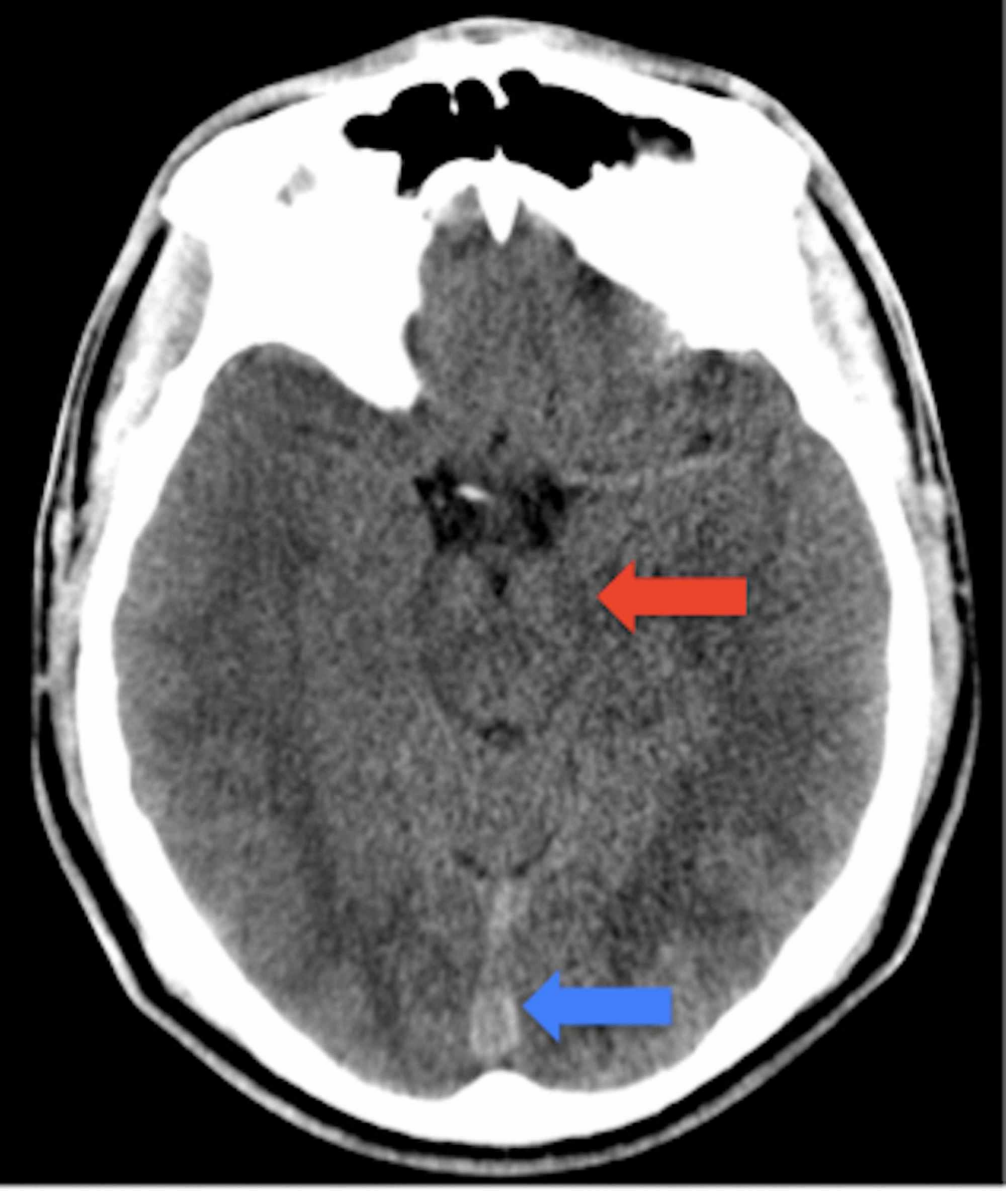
Noncontrast axial CT scan of the head at the level of the suprasellar cistern Mild effacement of crural cistern (red arrow), Prominence of straight sinus (blue arrow).
